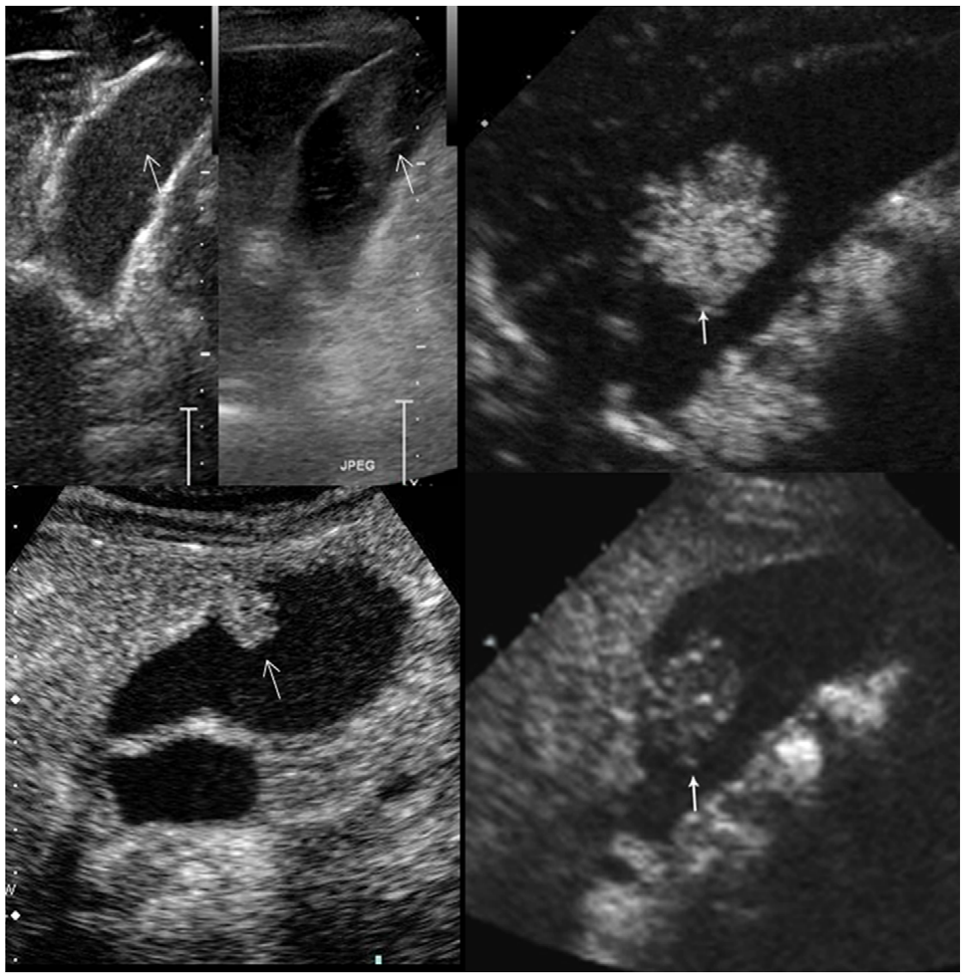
Enhancement extent of the gallbladder lesions on contrast-enhanced ultrasound. Upper left, non-enhancing; upper right, hyper-enhancing; lower left, iso-enhancing; lower-right, hypo-enhancing (arrows indicate the lesions).
